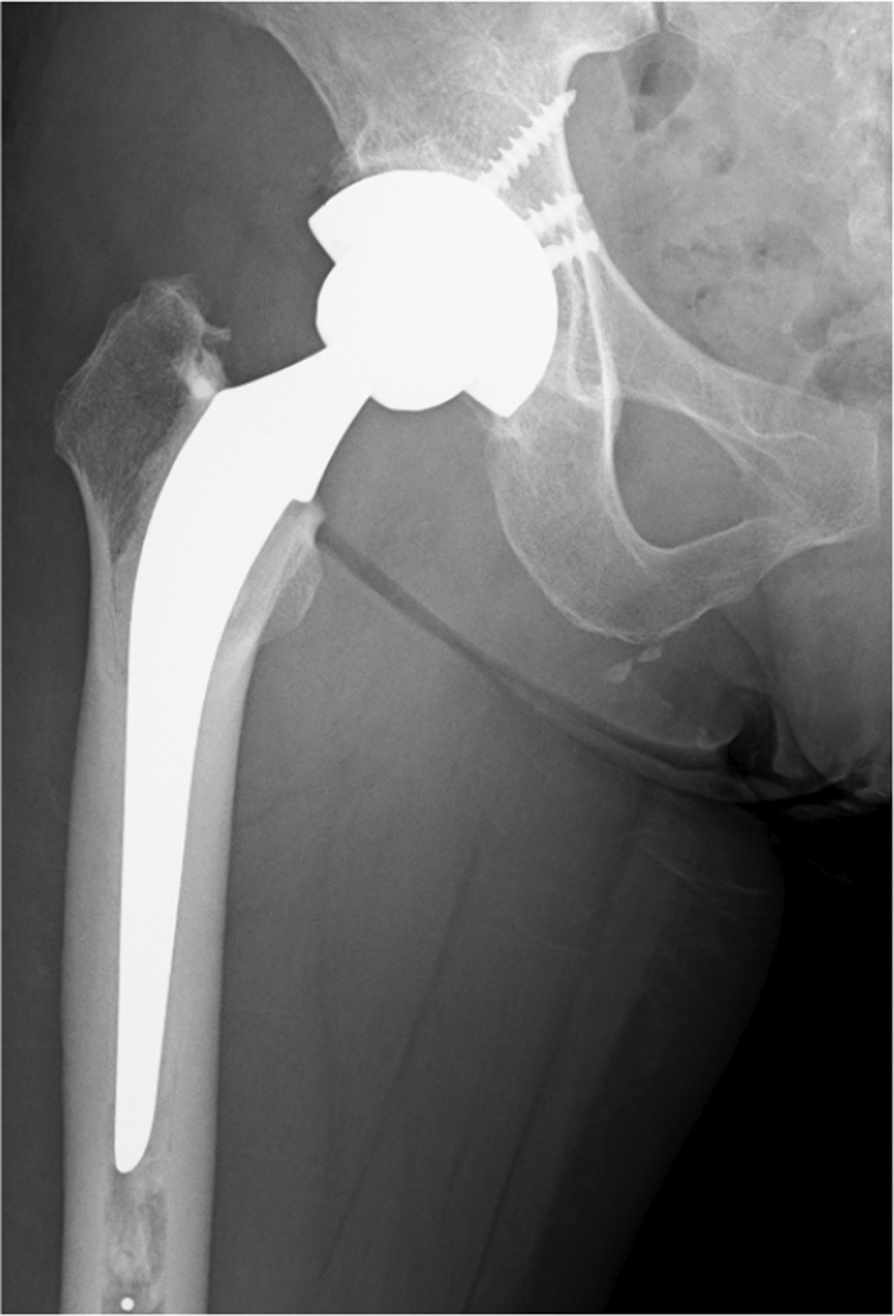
Radiograph after primary THA.
